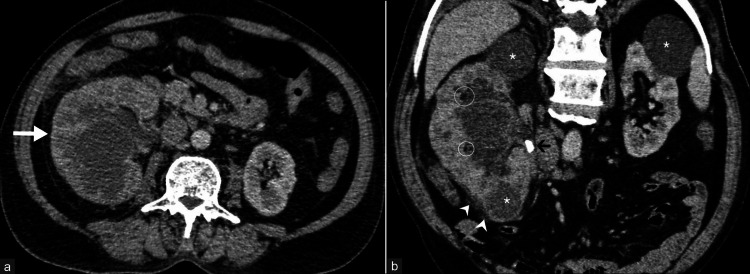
Xanthogranulomatous pyelonephritis with architectural distortion. A case of xanthogranulomatous pyelonephritis was diagnosed in a 70-year-old man. (a) Nephrographic phase axial CT urogram image shows grossly enlarged right kidney with gross pelvicalyceal dilatation. (b) Coronal reformatted image in nephrographic phase shows the architectural distortion of the right renal parenchyma and areas with fat attenuation foci (circles) with reduced enhancement compared to the left kidney, indicating declining renal function. Perinephric fat stranding (arrowheads) and the thickening of the right renal fascia are noted. An obstructive calculus (black arrow) is seen in the right pelviureteric junction. Multiple well-defined non-enhancing hypodense cortical cysts are seen in both kidneys (white asterisks). These images are from the hospital and have not been published elsewhere. CT: computed tomography
